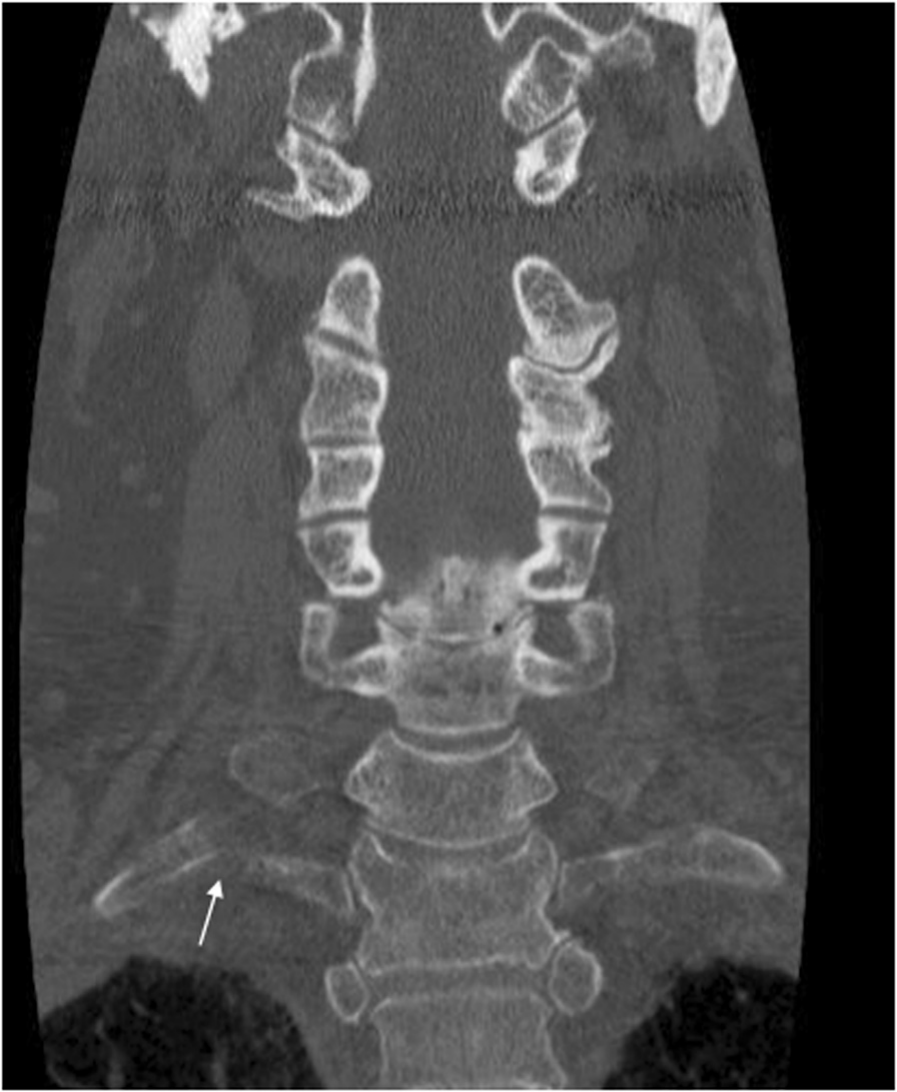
Non-contrast CT of the cervical spine. This coronal view reveals a displaced fracture of the proximal right first rib (white arrow).
